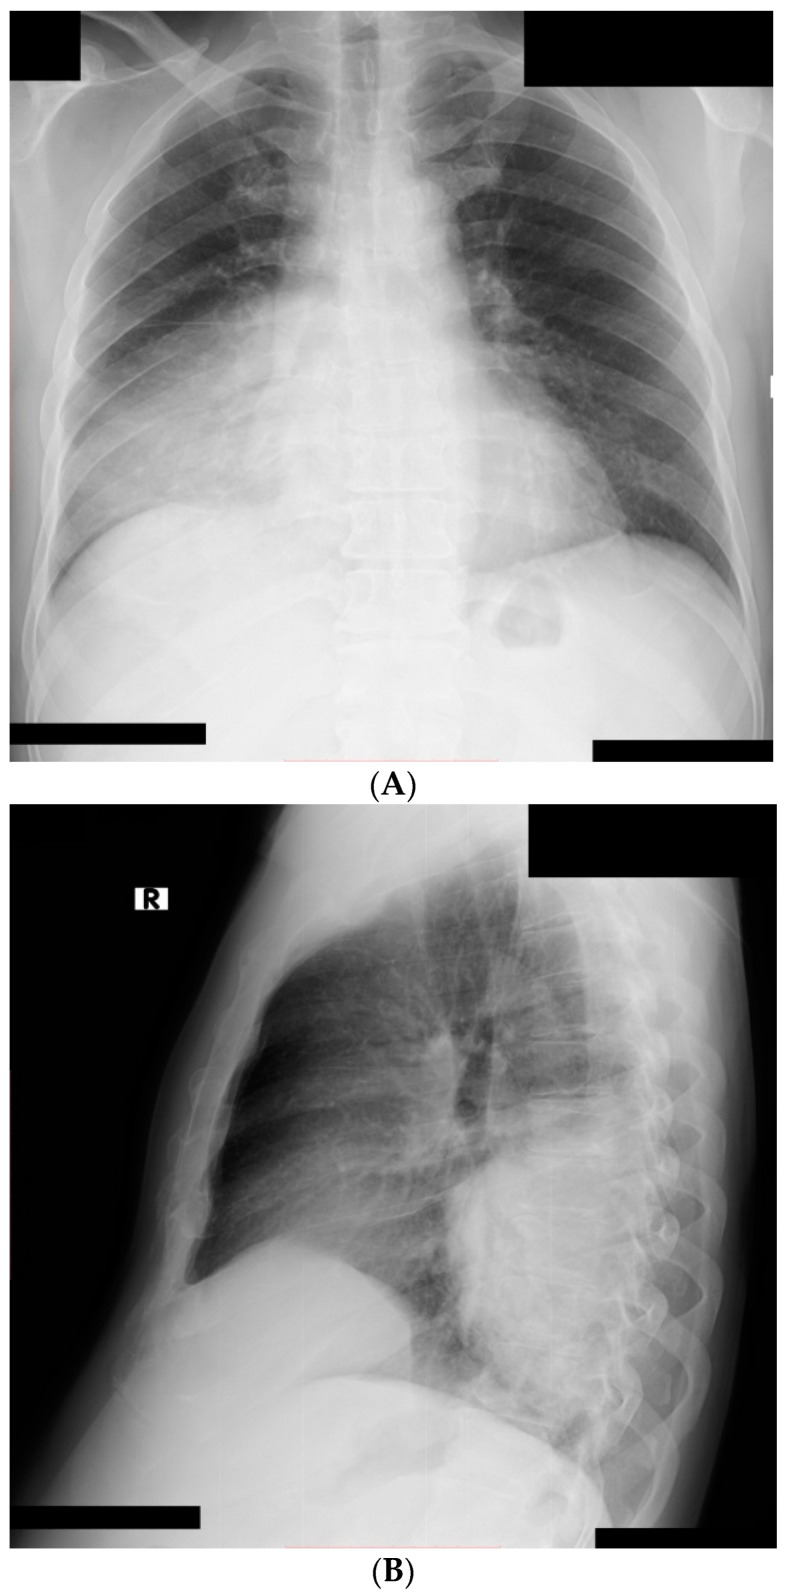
Chest X-ray: right pulmonary condensation syndrome ((A) postero-anterior view; (B) latero-lateral view).
